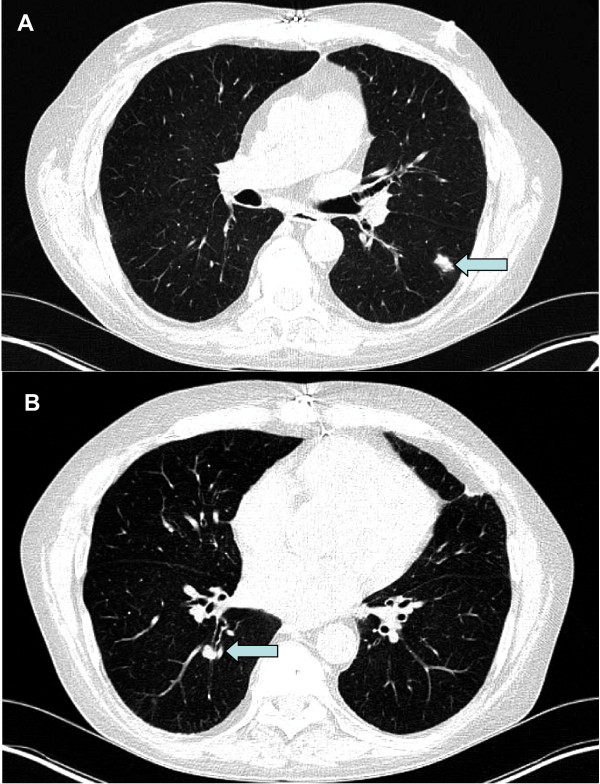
Lung imaging. Computed tomography displaying the progressively growing tumors (arrows) in the left (A) and right (B) lung.
